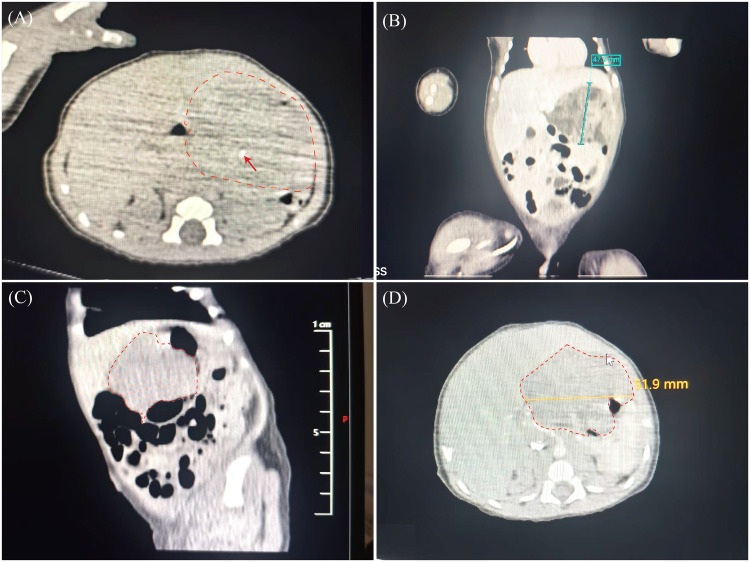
Preoperative abdominal computed tomography (CT) findings of a neonatal gastric teratoma arising from the anterior gastric wall. (A) Unenhanced axial CT image reveals a heterogeneous mass in the hepatogastric space containing a 2.9 mm hyperdense calcific focus (indicated by the red arrow). (B) Coronal and (C) sagittal contrast-enhanced CT reconstructions show the close association between the mass and the anterior gastric wall. (D) Contrast-enhanced axial CT shows the tumor measuring 51.9 mm in maximum diameter.
